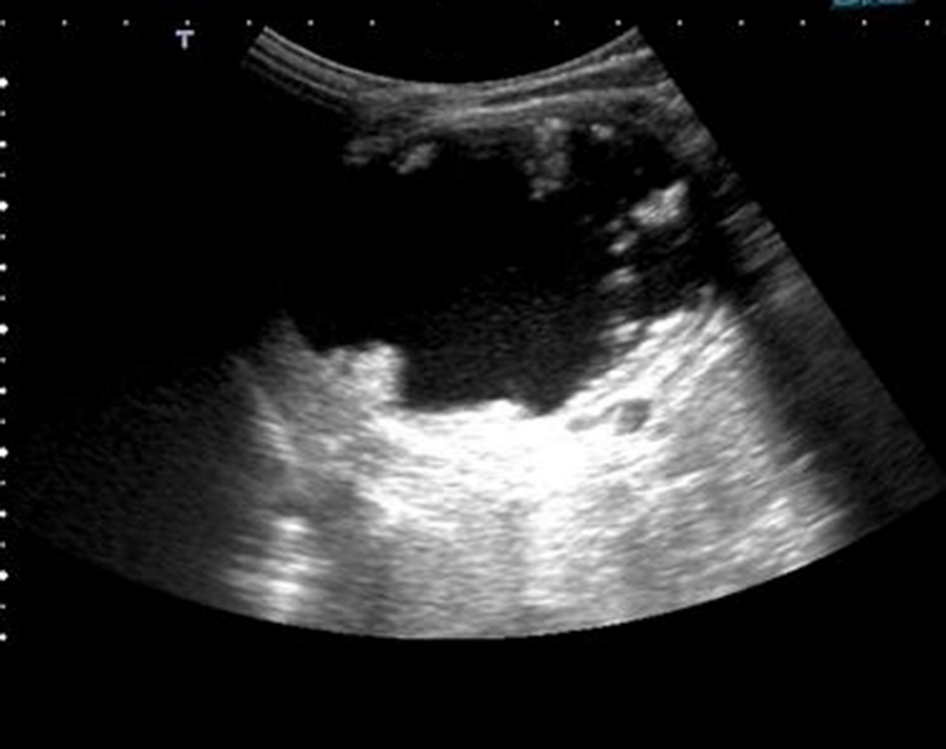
Sonographic posterior acoustic enhancement of the semi-solid cystic mass is clearly seen.
